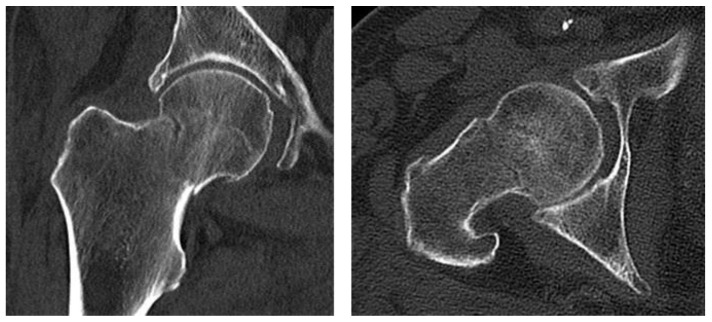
Computed tomography of the right hip reveals the femoral neck fracture. Considering the ultrasound finding and the clinical symptoms, a CT exam of the hip was performed, which revealed a femoral neck fracture. Due to the unquestionable nature of the image, we did not consider MRI for further diagnosis. The patient was then scheduled for a hemiarthroplasty of the right hip joint in the department of orthopedic surgery due to the old age of the patient. Ultrasound is not the primary diagnostic method for discovering hip fractures. Despite that, numerous studies and case reports have shown its reliability and potential in diagnosing occult femoral neck fractures where CT and X-ray fall short [14]. REMS is an accurate non-ionizing method for measuring the bone density at axial skeleton sites, and its results highly correlate to those measured using DEXA [2]. Moreover, the use of sonography to visualize and identify the ROI of the hip neck provides the opportunity to identify occult fractures. Such fractures are often hard to diagnose and missed by practitioners due to their atypical clinical and radiographic manifestation [15]. Fractures of the proximal femur are a common type of fragility fractures [16]. The ultrasound image of occult fractures may not always appear clearly as a break of the bone cortex, but also as an interference or abnormality of the surrounding tissue. The scan of the other hip is useful as a comparison. In addition, the joint could be examined for effusion. [17] The gold standard for diagnosis when plain radiographs and CT yield negative results is the MRI exam [18]. This method is costly and not highly available. On the other hand, bone density measurements such as REMS are carried out regularly and performed by wide variety of specialists.
